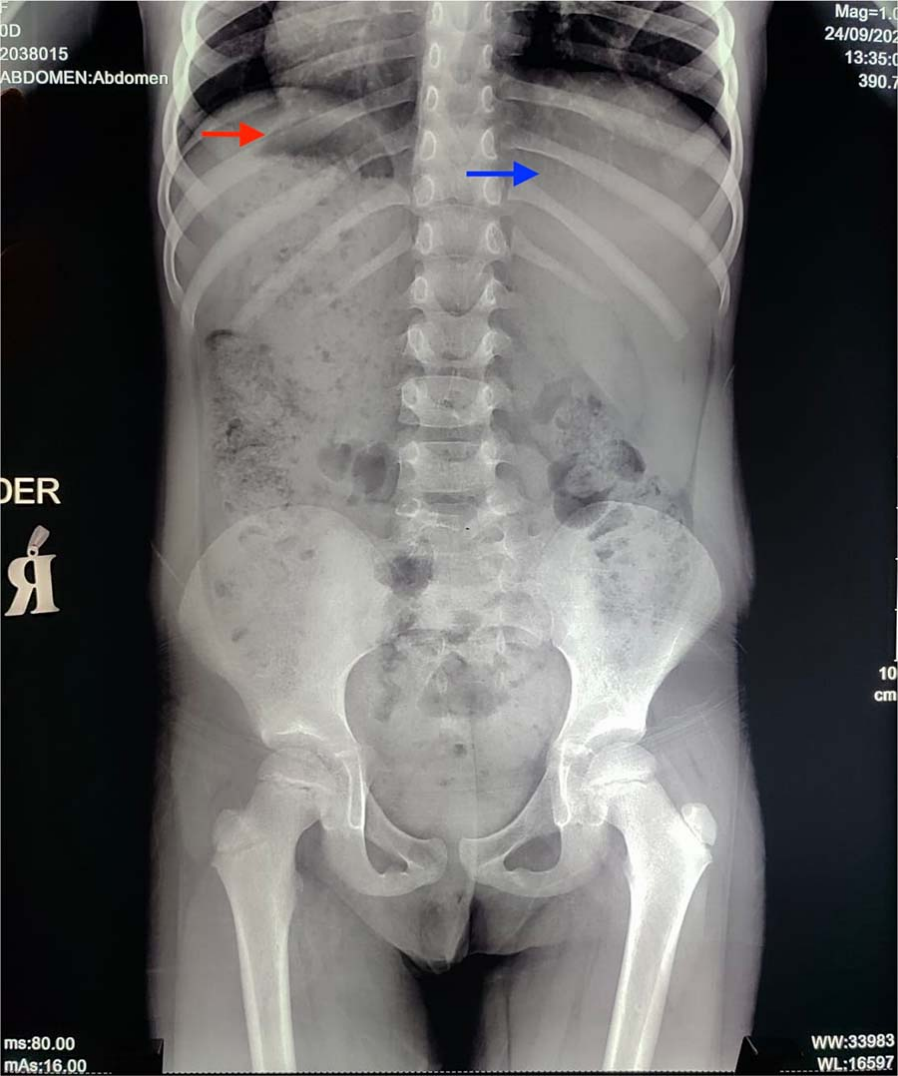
Abdominal radiograph. Consistent with left hepatic shadow (blue arrow) and right gastric bubble (red arrow).
